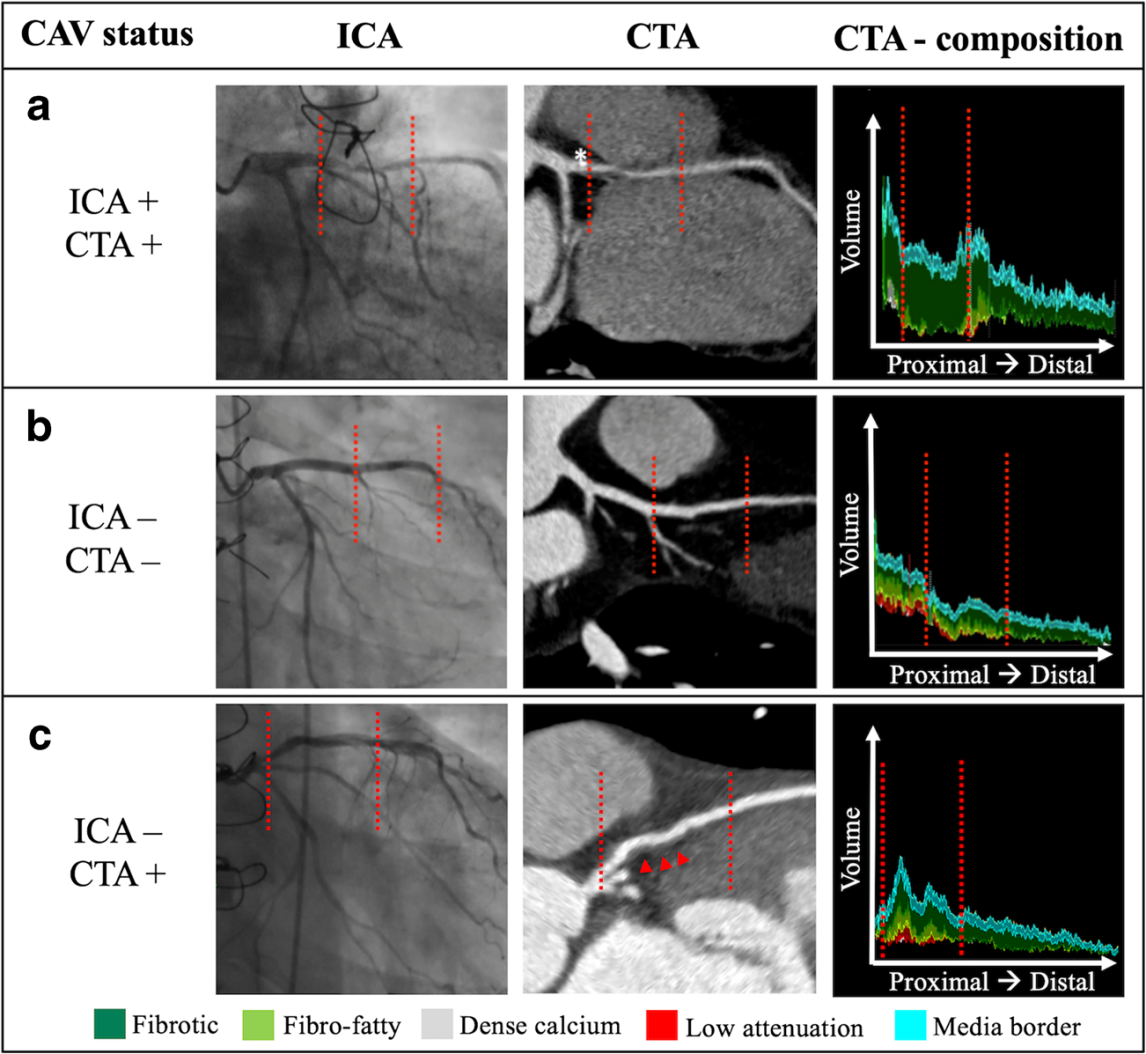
Imaging examples stratified by CAV status. Left coronary arteries of three patients with (a) CAV on ICA and CTA, corresponding luminal stenoses on ICA and wall thickening on CTA; (b) patient without signs of CAV, with normal coronary lumen on ICA and thin coronary wall on CTA; and (c) patient with normal coronary lumen on ICA (also no stenosis on CTA), but wall thickening (red arrowheads) on CTA. Red dotted lines mark the reference areas of interest. CAV, cardiac allograft vasculopathy; CTA, computed tomography angiography; ICA, invasive coronary angiography
